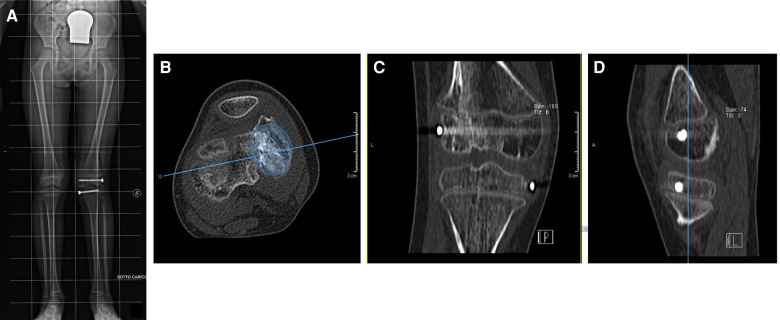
Four months postoperative x-ray showing a valgus (20°) of the left lower limb and the absence of dysmetria (A). CT scans confirm the presence of femoral bone bridge in three different projections (B–D). (B) shows that the bony bar is 30% of the entire area of the growth plate.
